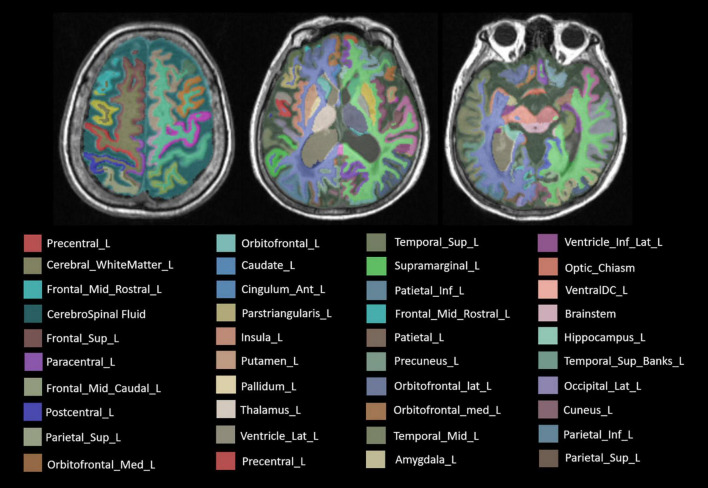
The label of main brain regions in structural MRI (sMRI) after automatic segmentation. Specially, the symmetrical structure is divided into left and right and has different labels. Only the structure on the left is marked above.
